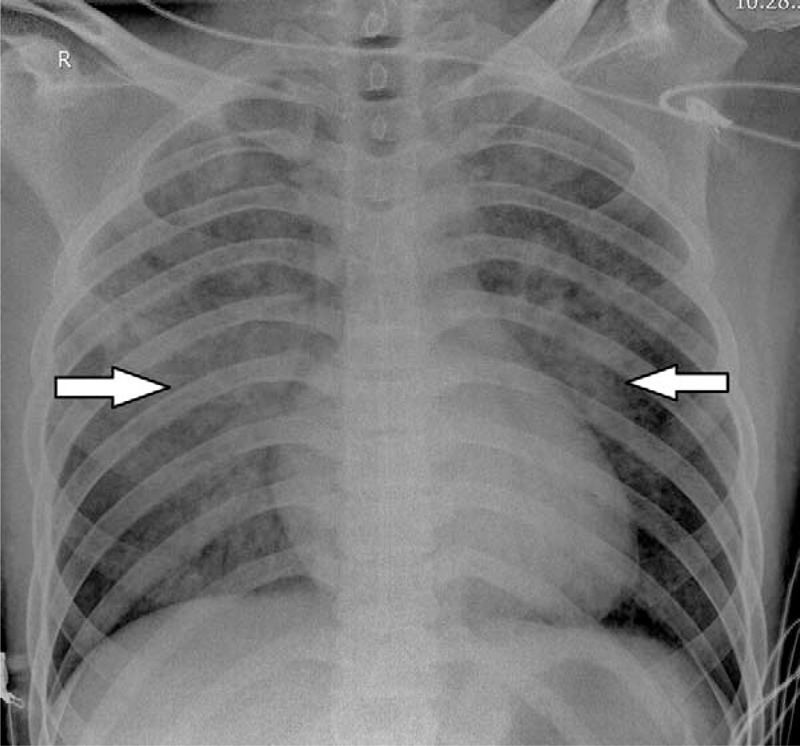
Chest x-ray in 1 hour after ROSC showed a hazy opacification spreading from the lung hilar regions (arrows). ROSC = return of spontaneous circulation.
